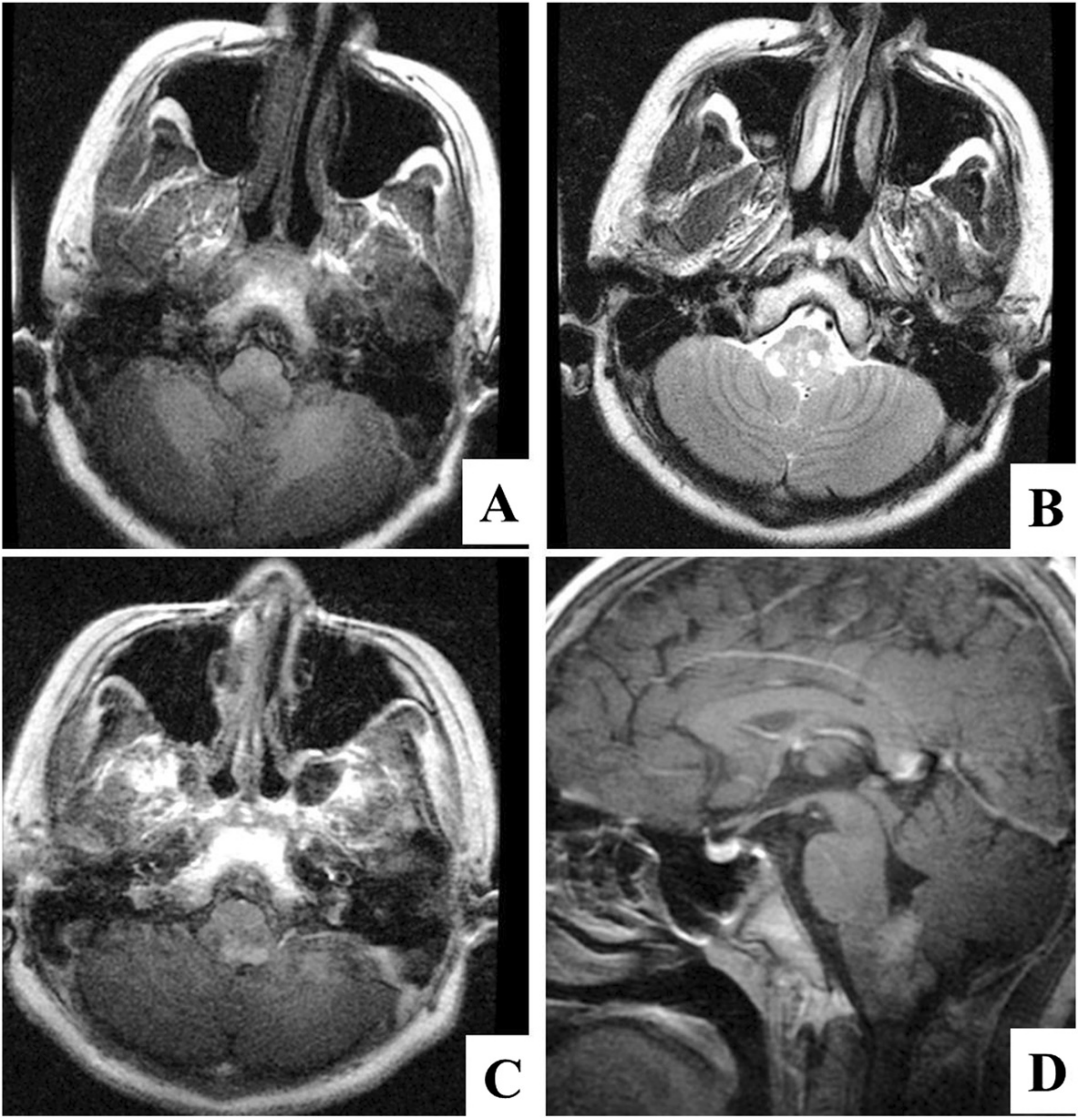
Magnetic resonance imaging (MRI) scans of the case. Axonal brain MRI shows a lesion in the dorsal medulla oblongata with low T1-weighted (A) and high T2-weighted signals (B). Contrast-enhanced axial (C) and sagittal (D) T1-weighted images show the contrast-enhanced lesion.
